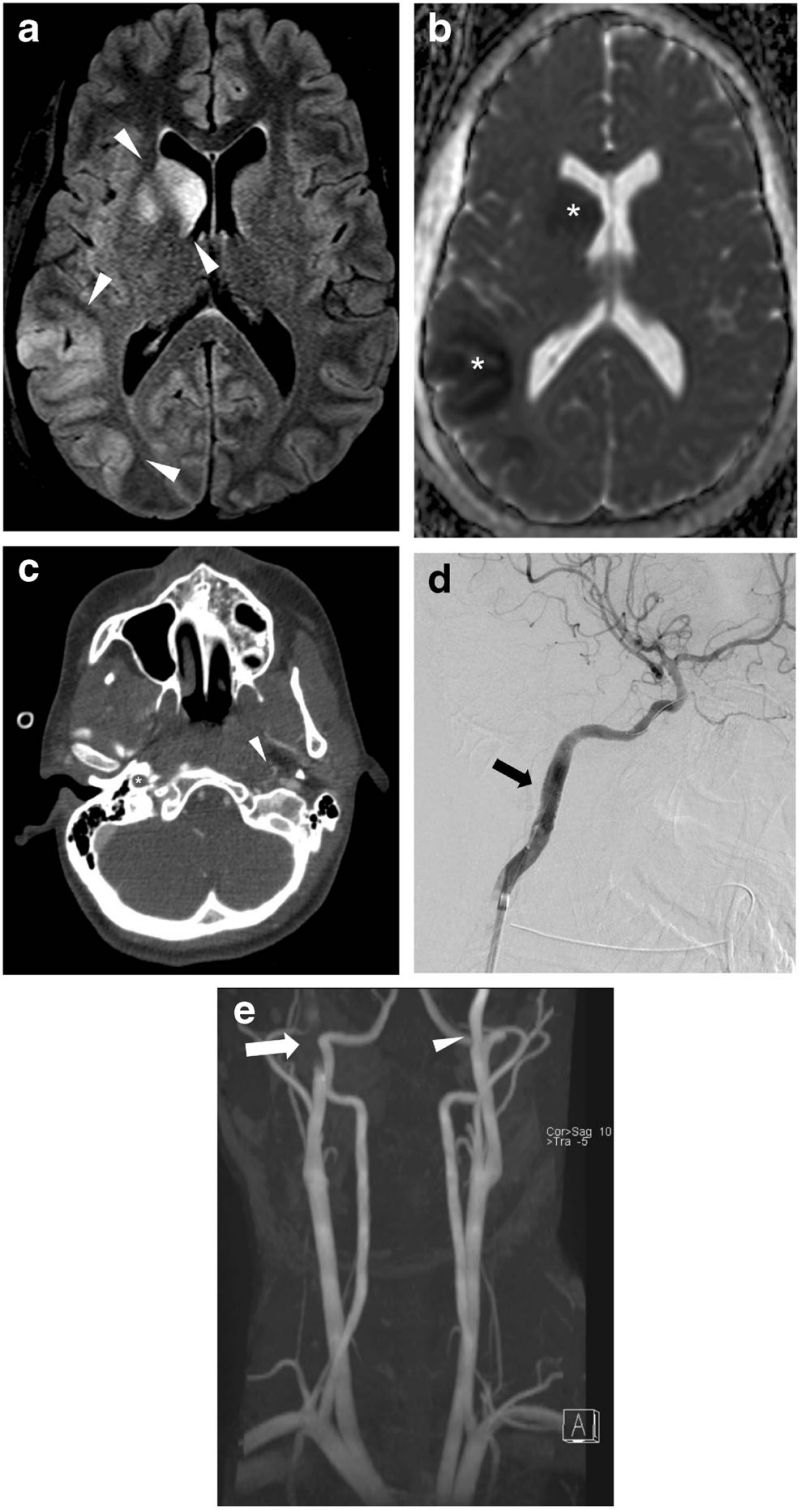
Twenty one-year-old female with polytrauma after MVA; BCVI of both ICA. a Ischemic lesion and focal swelling in the area of the right caudate nucleus and lentiform nucleus, with an additional ischemic lesion in the right parietal cortex (1.5 T MRI; FLAIR). b Both lesions show decreased diffusion (arrowheads; 1.5 T MRI; DWI). c Grade IV BCVI of the right ICA (asterisk), and grade II lesion of the left ICA on the level of the skull base (arrowhead) on axial CTA images. Both VA remain open. d Wire stent of the right ICA placed under fluoroscopy guidance on DSA summation image (arrow). e 1.5 T MRA of the craniocervical arteries after stent placement demonstrating near normal patency. Signal loss caused by the metallic wire stent (arrow) in the right ICA. Residual grade II BCVI lesion of the left ICA (arrowhead)
